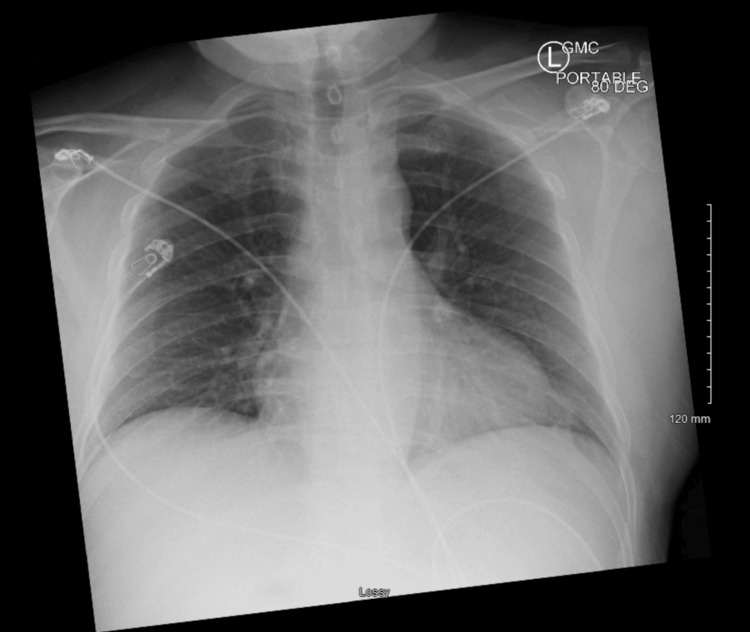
CXR in ED CXR obtained in ED showing mild diffuse interstitial opacities likely representing atelectasis secondary to low lung volumes CXR: chest x-ray; ED: emergency department
